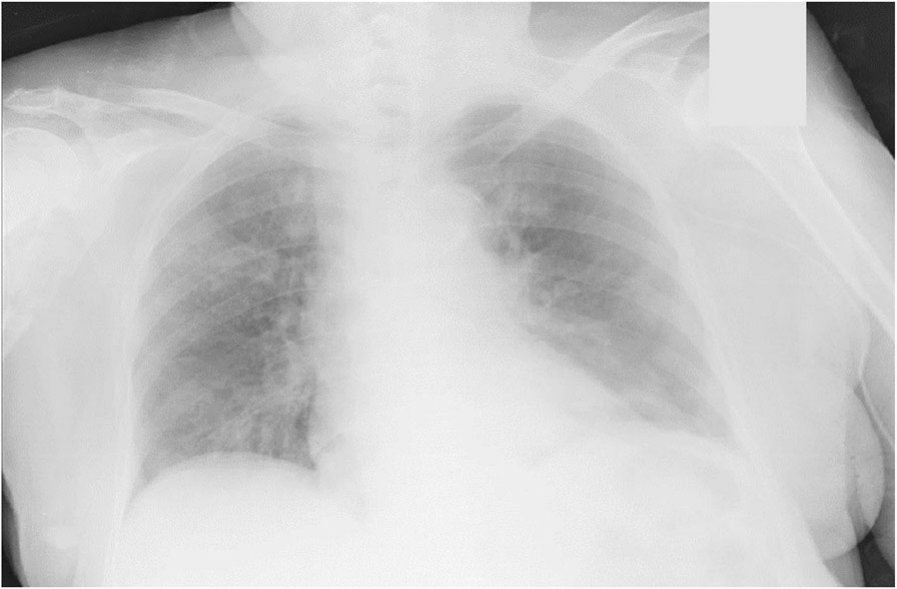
Chest X-ray performed on admission (single supine anteroposterior view) showing bilateral patchy shadowing
